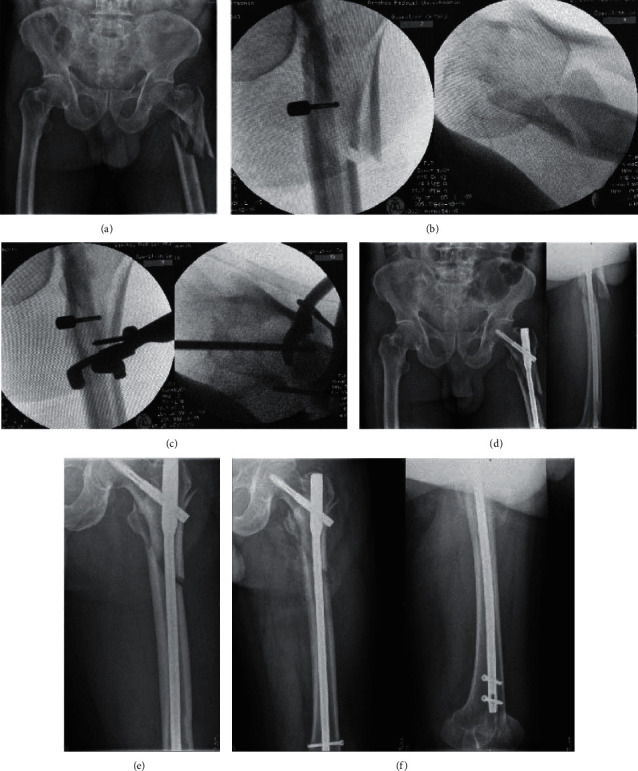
Preoperative X-ray of the patient, Seinsheime classification V (a). During the operation, the length of the fracture was restored by traction with the retractor, and the displacement of the fracture block was obvious (b). By the minimally invasive way, the reduction forceps are used to reduce the fracture piece from the front and the outside to achieve anatomical reduction as far as possible (c). X-ray examination was performed 2 days after surgery. It showed that the fracture piece was displaced, which proved that the fracture piece was unstable (d). Two months after the operation, it was found that there was no callus formation at the fracture site, and the fracture line was still clear (e). Six months after the operation, it was found that a large number of calluses formed at the end of the fracture, and the fracture healed well (f).
